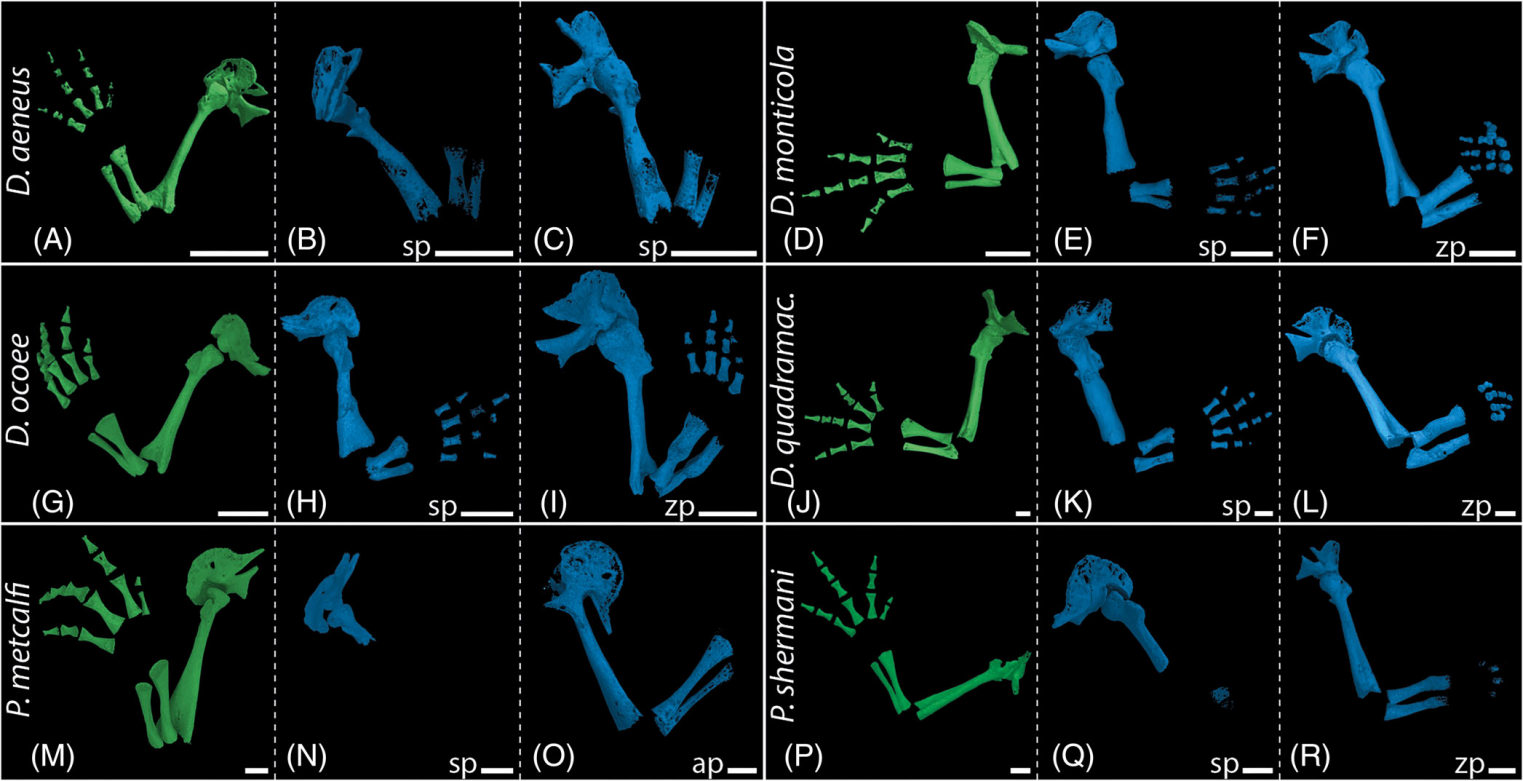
In‐lab amputations and outcomes of long‐term fore limb regeneration. Segmented 3D models of unstained micro‐CT scans showing bulky long bones after regeneration. *Desmognathus aeneus*: Representative image of a normal, non‐regenerated limb (A). Regenerated limbs after amputation at the humerus (B and C). *Desmognathus monticola*: Representative image of a normal limb (D). Regenerated limbs after amputation at the humerus (E) and radius/ulna(F). *Desmognathus ocoee*: Representative image of a normal limb (G). Regenerated limbs after amputation at the humerus (H) and radius/ulna (I). *Desmognathus quadramaculatus*: Representative image of a normal limb (J). Regenerated limbs after amputation at the humerus (K) and radius/ulna (L). *Plethodon metcalfi*: Representative image of a normal limb (M). Regenerated limbs after amputation at the humerus (N) and carpal bones (O). *Plethodon shermani*: Representative image of a normal limb (P). Regenerated limbs after amputation at the humerus (Q) and radius/ulna (R). Green color represents contralateral unamputated control limbs, blue color represents regenerated limbs. ap, amputation site autopod; sp, amputation site stylopod; zp, amputation site zeugopod. White scale bars represent 1 mm.
